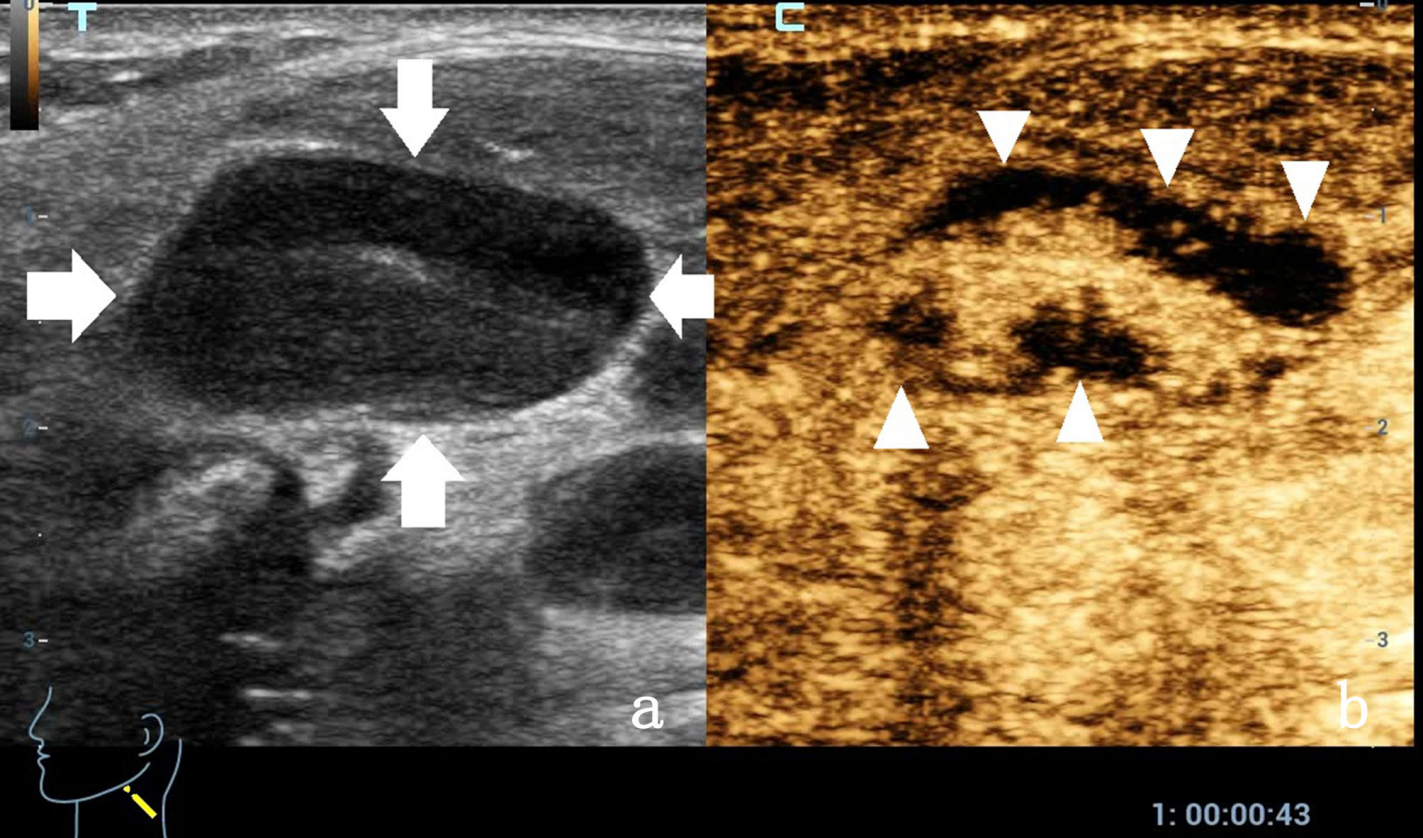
A 33-year-old man with enlarged lymph nodes in his left neck. (a) The conventional ultrasound control image shows enlarged lymph nodes with obvious cortical thickening and uneven internal echo. Arrows showing enlarged lymph nodes. (b) Contrast-enhanced ultrasound showing an irregular nonenhanced area (arrow head) in the lymph nodes, which was later confirmed as a necrotic area by core needle biopsy.
